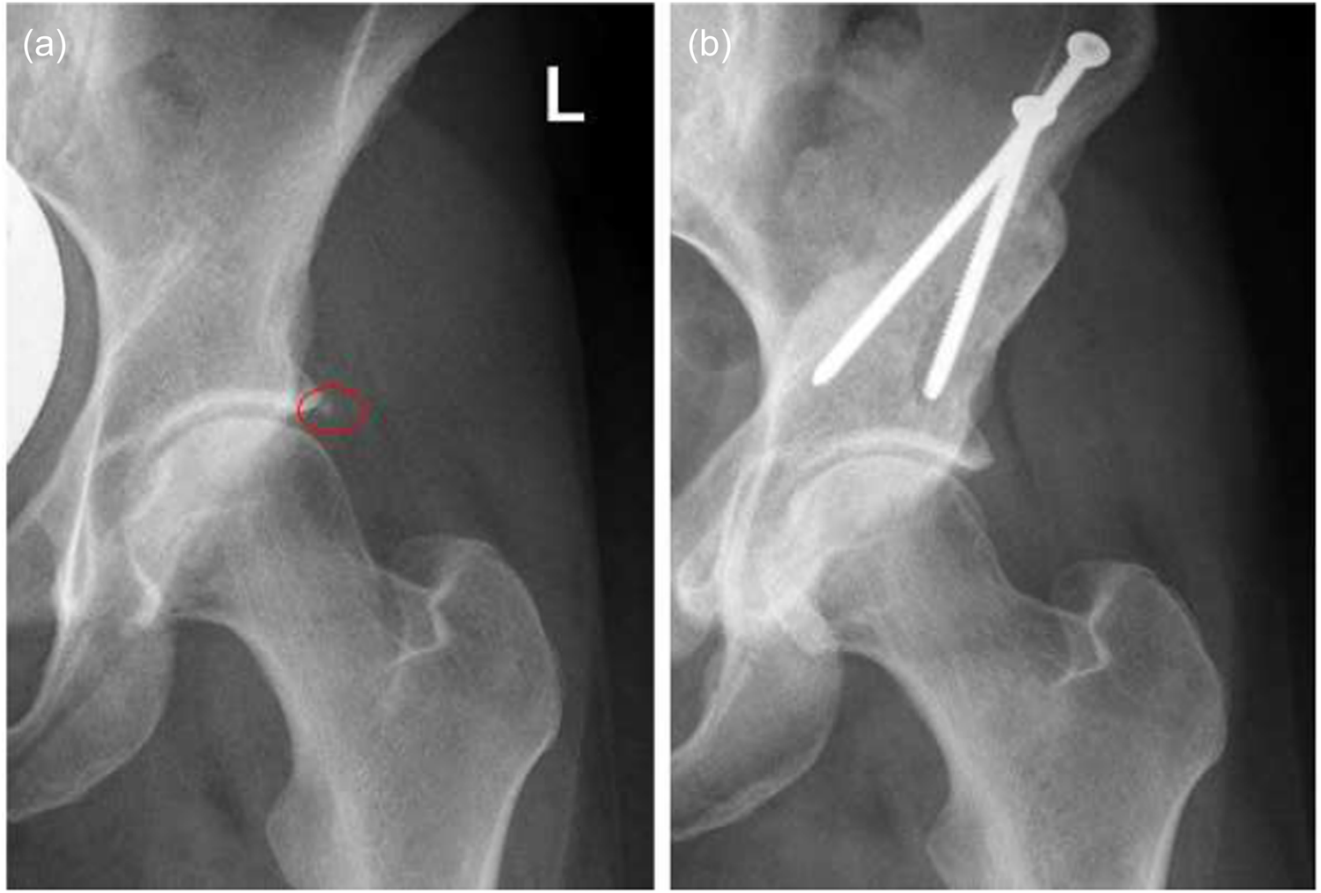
Anteroposterior pelvic radiographs of a borderline dysplastic hip showing labral ossification preoperatively (a, left hip; red circle) and complete remodellingat 1‐year follow‐up after isolated periacetabular osteotomy (b).
